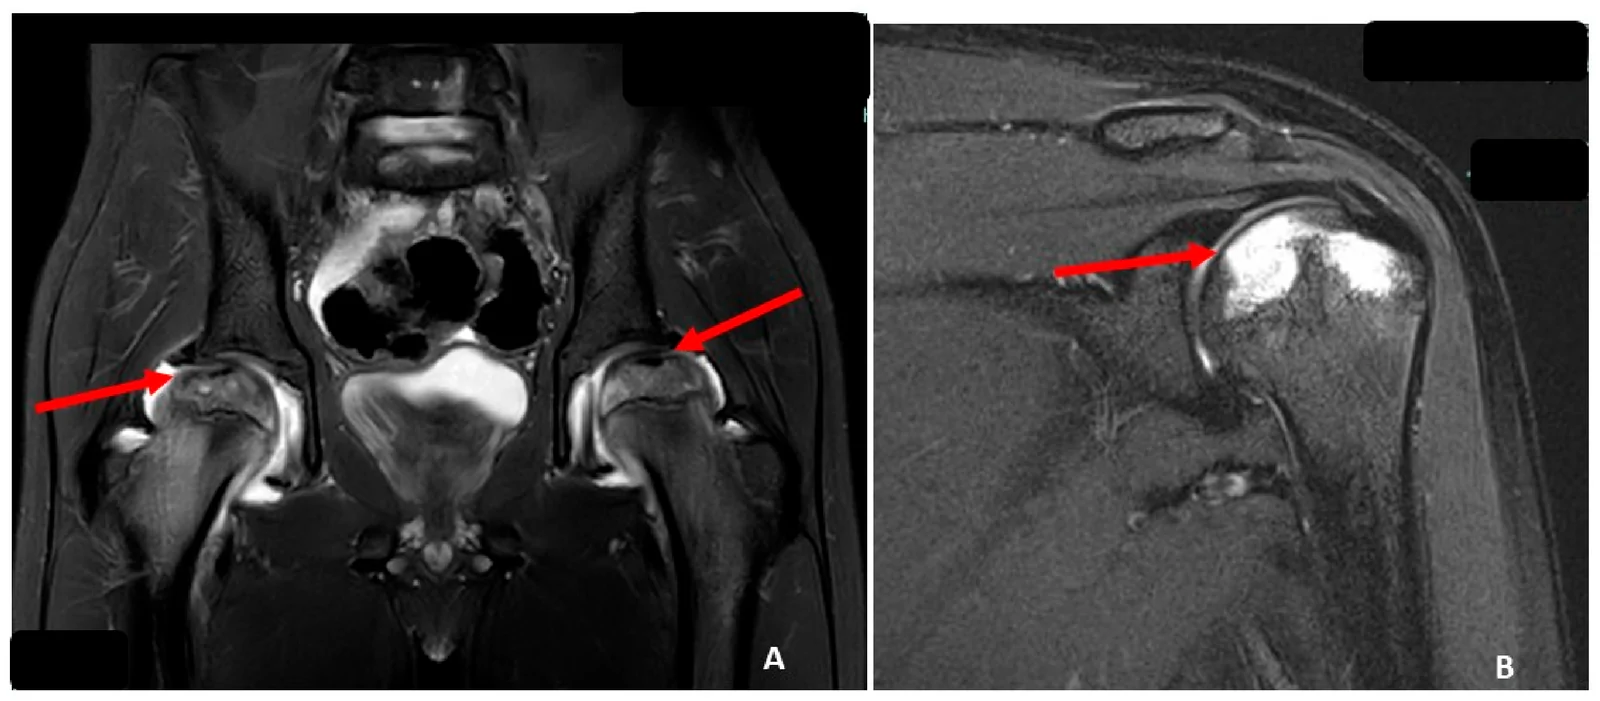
Osteonecrosis involving the articular surfaces of long bones in children with sickle cell disease. Panel (A) shows MRI findings of early osteonecrosis involving both femoral heads (red arrows) in a 12-year-old child. Panel (B) demonstrates osteonecrosis of the left humeral head (red arrow) in a 14-year-old child with sickle cell disease.
